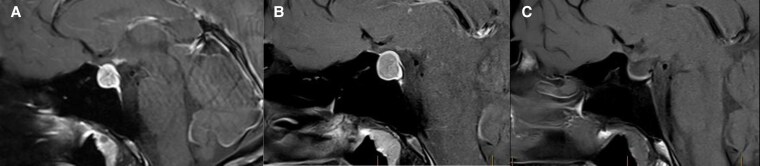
Figure depicts a series of postcontrast T1 MRI images in the sagittal plane showing the evolution of the pituitary lesion. The left-most picture shows the macroadenoma at time of diagnosis in January 2023. The middle image shows the interval increase in size in August 2023. The image on the right side demonstrates complete resolution of the image by February 2024 with possible interval development of a partial empty sella.
